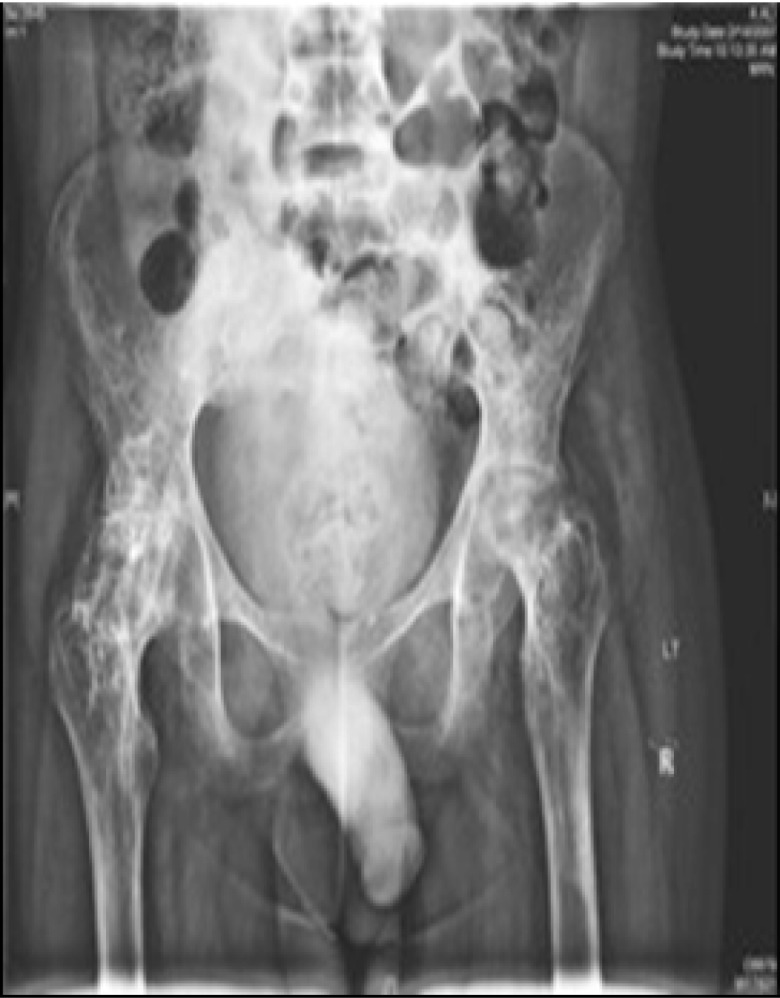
AP Hips x-rays showed severe avascular necrosis and ankylosis.
